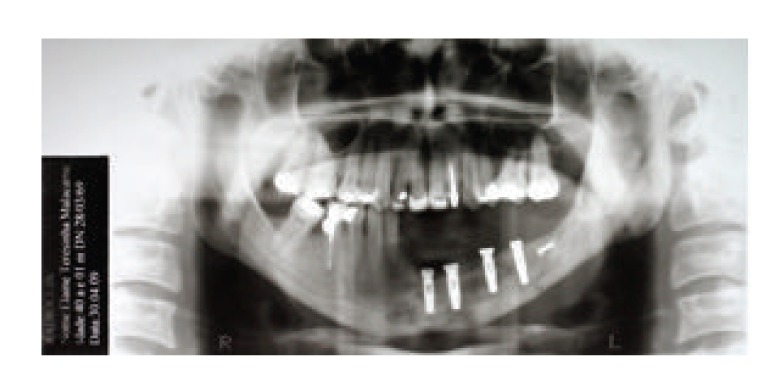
Panoramic radiographic showing implants placed in position.
